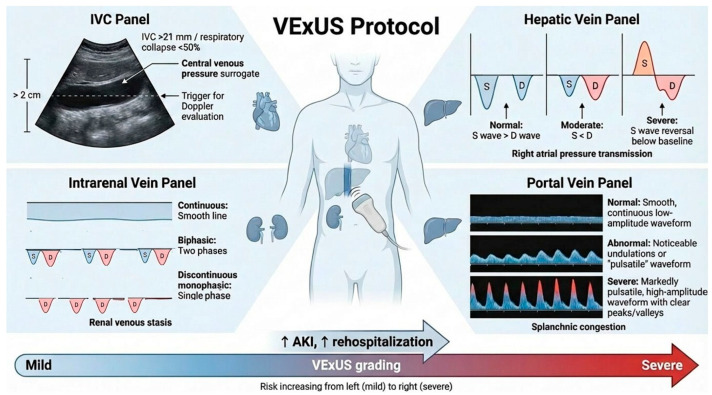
VExUS protocol and physiological interpretation. The VExUS score integrates IVC morphology with Doppler assessment of hepatic, portal, and intrarenal veins. Progressive waveform abnormalities reflect increasing venous pressure transmission and organ-level congestion. Higher VExUS grades are associated with increased risk of organ dysfunction, particularly acute kidney injury. VExUS reflects hemodynamic congestion rather than intravascular volume alone. Abbreviations: VexUS, venous excess ultrasound; IVC, inferior vena cava; S, systolic; D, diastolic; AKI, acute kidney injury.
